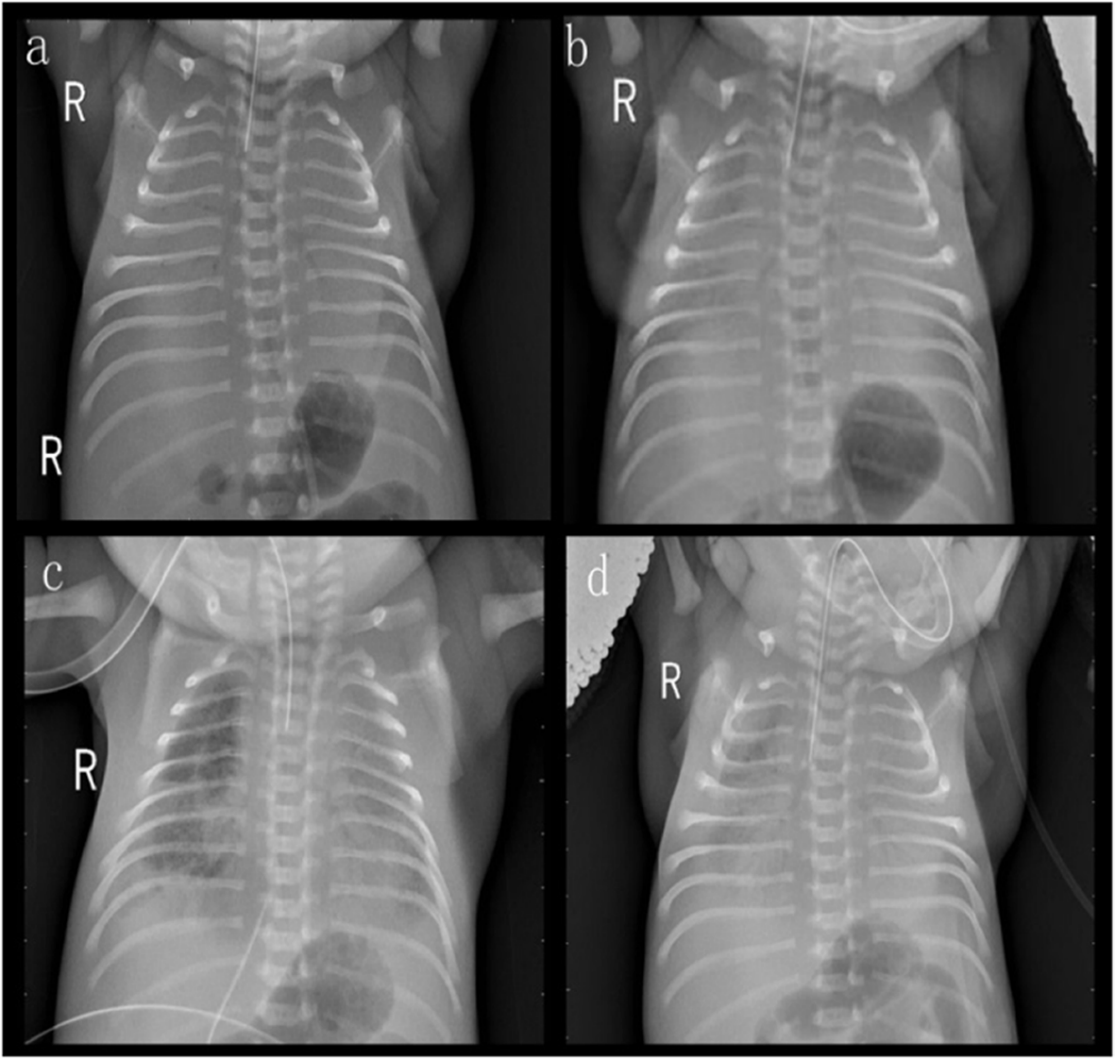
Chest X-ray before treatment documented normal ribs and vertebrae, and the transmittance of both lungs decreased significantly, leading to a presentation of white lung (a). After 1 round (b), 2 rounds (c) and 3 rounds (d) of pulmonary surfactant therapy, chest X-ray showed white lung
